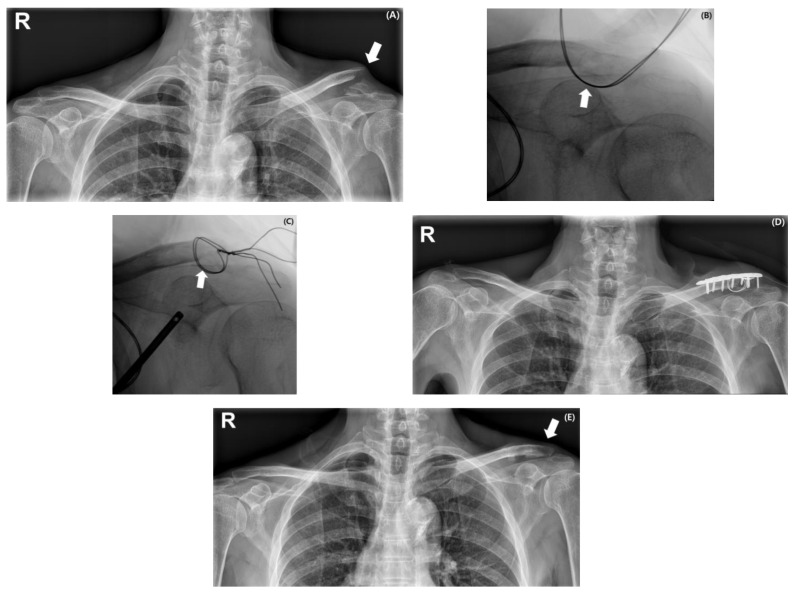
Serial images of a 63-year-old man with a type IID distal clavicle fracture following a fall from height. Preoperative radiograph shows a displaced fracture of the distal clavicle with a prominent medial fracture beak causing skin tenting (indicated by a white arrow) (A). Intraoperative fluoroscopic images demonstrate fracture reduction in the inferior fragment achieved using cerclage wiring (indicated by a white arrow) (B,C). Immediate postoperative radiograph confirms appropriate reduction and stable fixation using a locking plate with cerclage wiring (D). At 15 months after implant removal, follow-up radiograph shows maintained reduction and complete healing without complications (indicated by a white arrow) (E).
